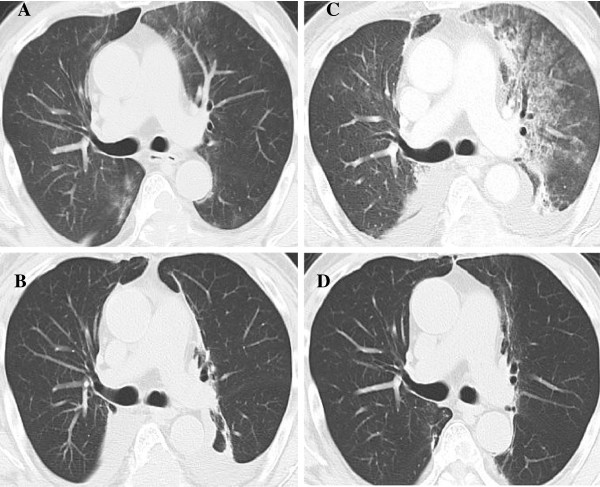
Radiation recall pneumonitis induced by sunitinib. On the 14th day of sunitinib induction, bilateral lung consolidation appeared (A). During follow-up without sunitinib, consolidation developed along with progressive fever (B). After steroid pulse therapy, the CT findings revealed that the interstitial filtration was localized in the previously irradiated area (C). No evidence of relapse of recall pneumonitis during sorafenib treatment was observed (D).
